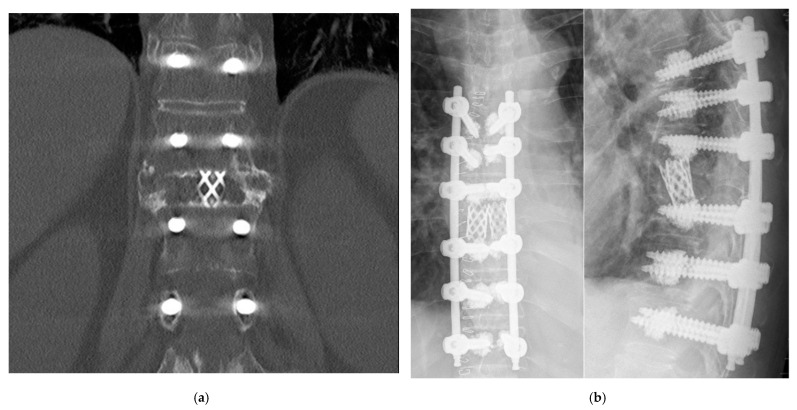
(a) Solid bony fusion. This figure shows the typical solid bony fusion between the cranial and caudal adjacent vertebral body, bridging the harms mesh. Usually, the bony fusion occurs bilaterally in a bracketlike manner. (b) Typical conventional X-ray after posterior only vertebral column resection in anteroposterior (left) and lateral plane (right): Augmented pedicle screws were inserted three levels above and below the target vertebra. The target vertebra was resected in a posterior-only approach. Harms meshes were inserted. The spine was relordosed and the affected segment compressed. Spondylodesis was established using autologous bone.
